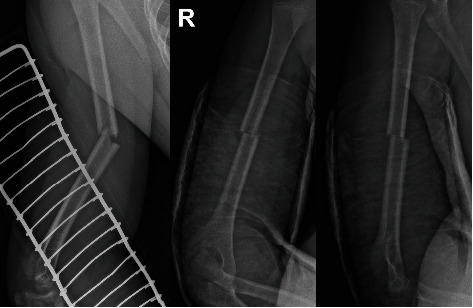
X-ray of the simple transverse humeral shaft fracture (12A3b), most often associated with radial nerve palsy in children (figures from the Department of Trauma and Orthopedic Surgery database, Upper Silesian Children's Health Center, John Paul, Katowice, Poland).
